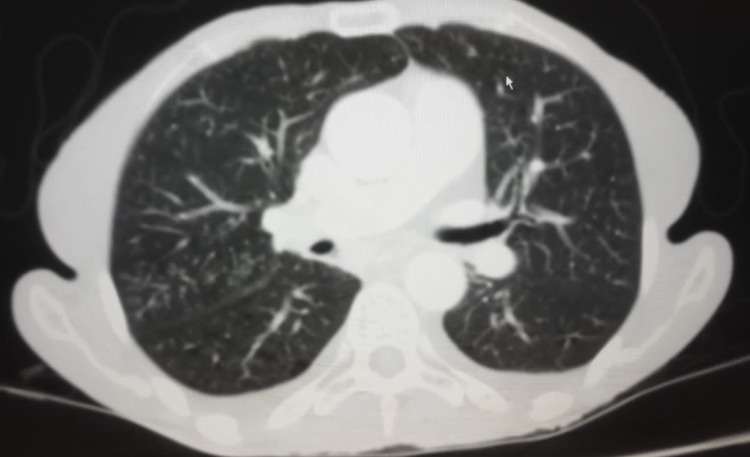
CT scan of the chest showing a tree in a bud appearance
